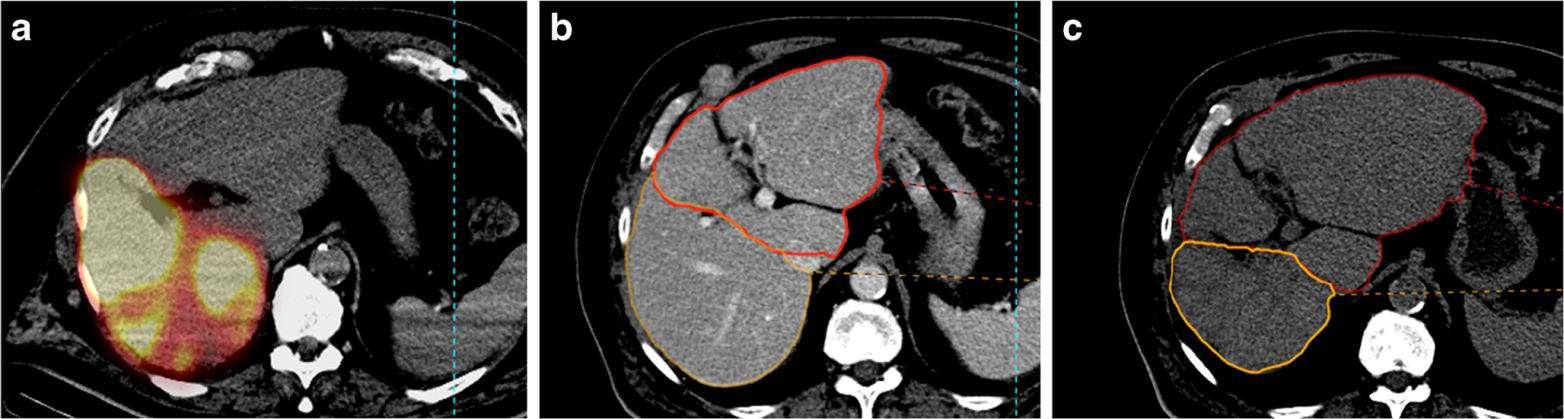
Example of hypertrophy related to a high HILD. This 74-year-old man with a Child A5 cirrhosis was treated with 2.2 GBq of ^90^Y–loaded glass microspheres injected in the RHA for HCC recurrences in the right lobe after chemoembolization. ILD was 121 Gy, HILD 114 Gy, and TD 346 Gy for a tumoral volume of 43cm^3^. The MAA scintigraphy showed an absence of perfusion of the segment 1 (a). The initial hepatic reserve was 52% (b). At 9 months, a hypertrophy of 66% of the left lobe (+ segment 1) was found and a 54% atrophy of the treated right lobe (excluding segment 1) was observed (C). The hepatic reserve after RE was 79.9%
